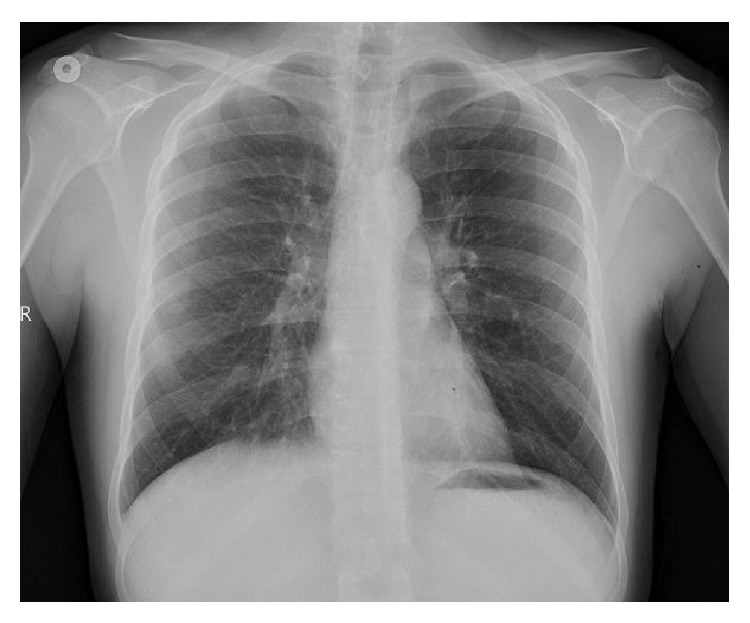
The chest X-ray of patient in the first month.
